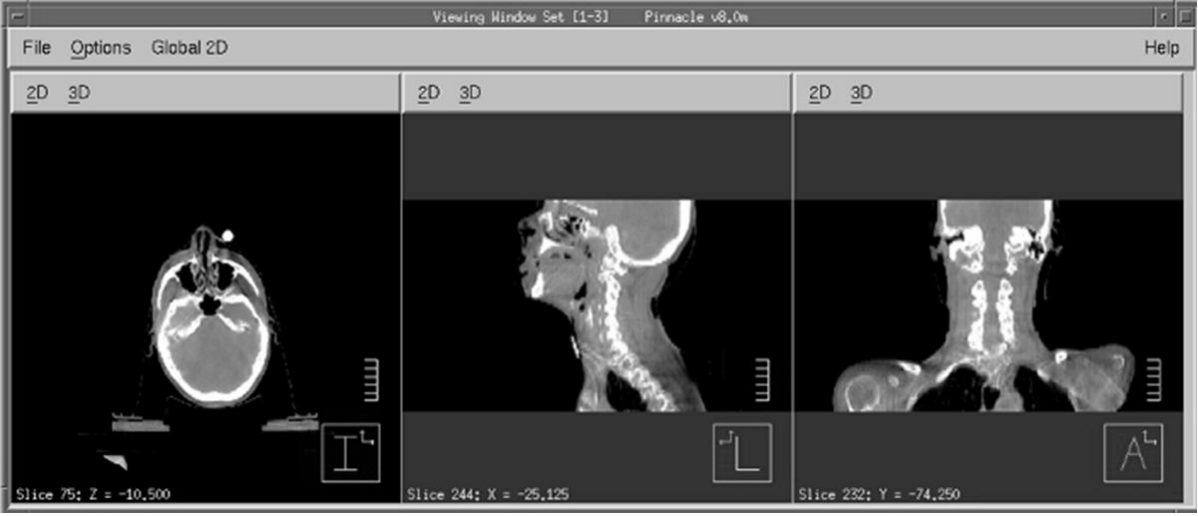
Combined double orbit CBCT images of an example head and neck patient imported into Pinnacle via DICOM.
